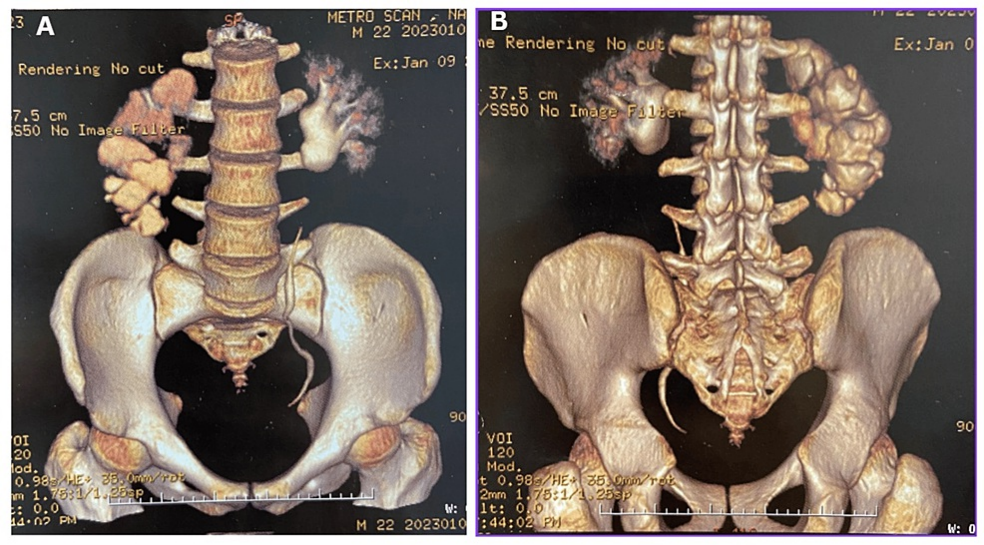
CT scan: anterior and posterior views of the right kidney CT: computed tomography
